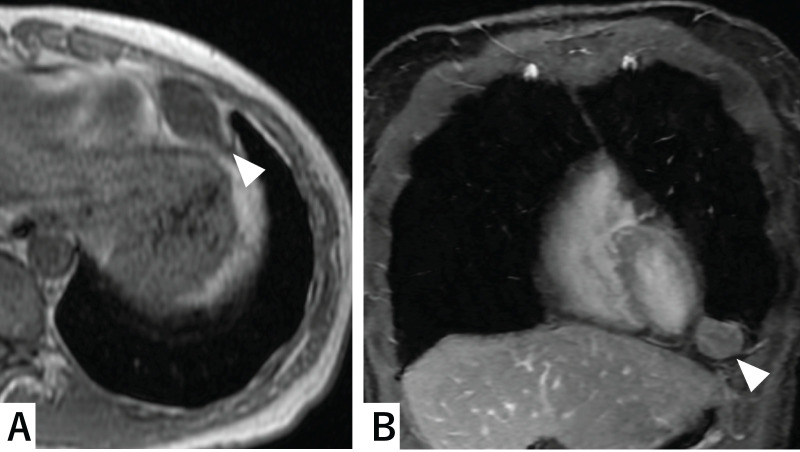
T2-weighted MRI showing a mildly high signal intensity (arrowheads). (A: axial, B: coronal).
